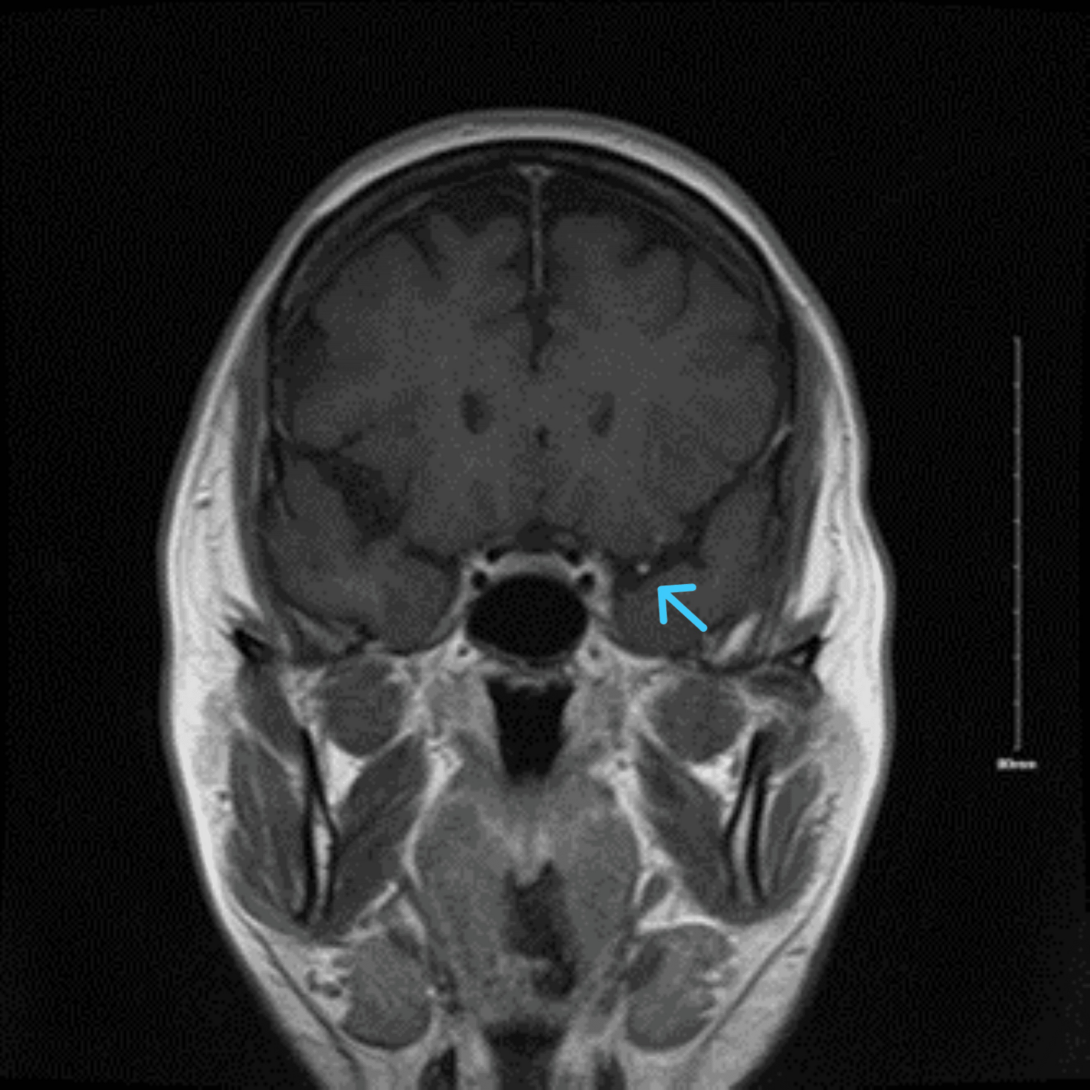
Left facial nerve enhancement on post contrast coronal magnetic resonance imaging
